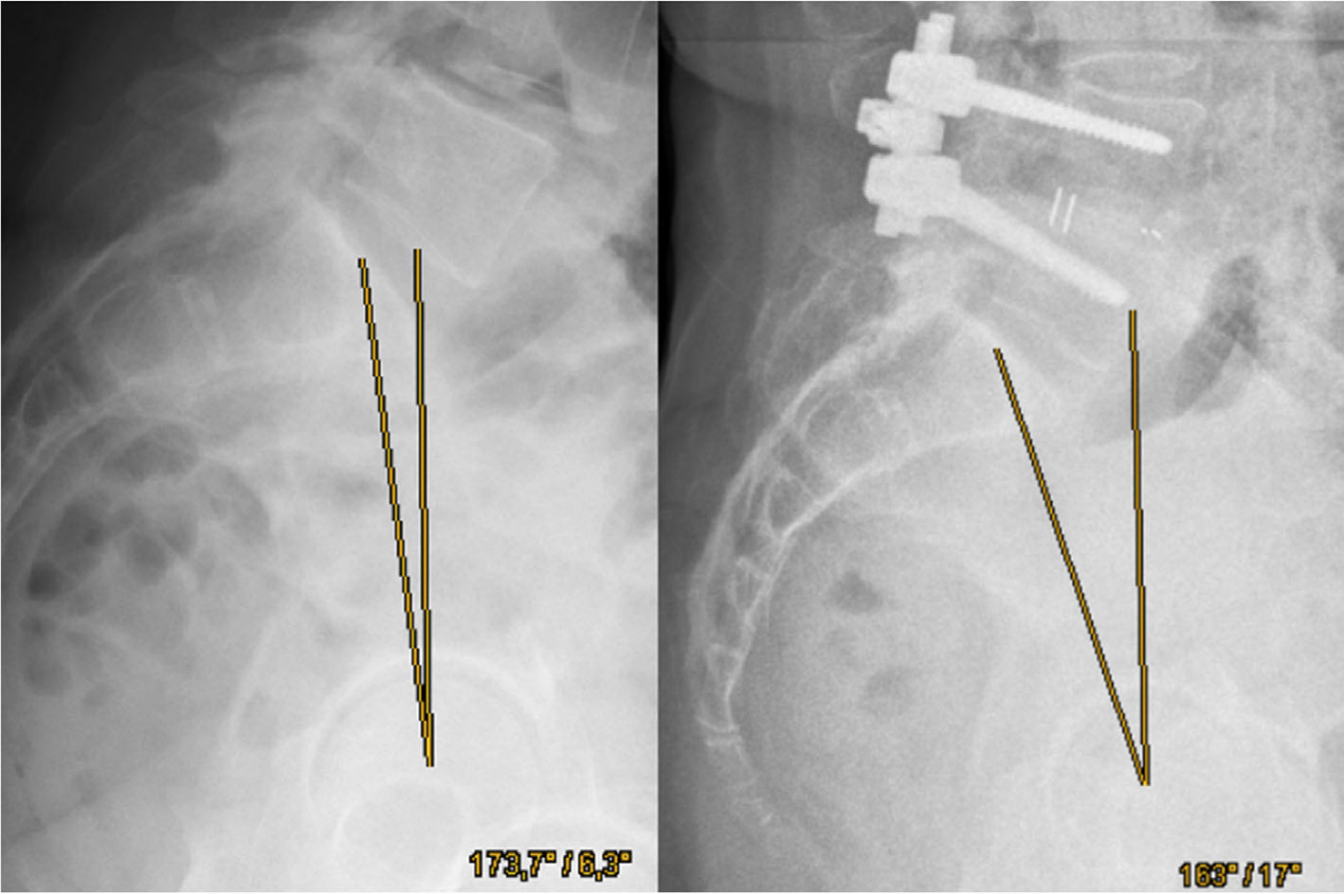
Pre‐ and postoperative (PLIF L4/5) lateral standing radiographs of the lumbar spine. The pelvic tilt is defined as the angle between the two depicted lines. The first line goes from the middle of the superior endplate of S1 to the middle of the femoral head and the second line is the vertical reference. The greater the difference of the pelvic tilt after fusion surgery compared to the preoperative value, the worse is the outcome after 6‐years when quantified by the EQ‐5D questionnaire.
